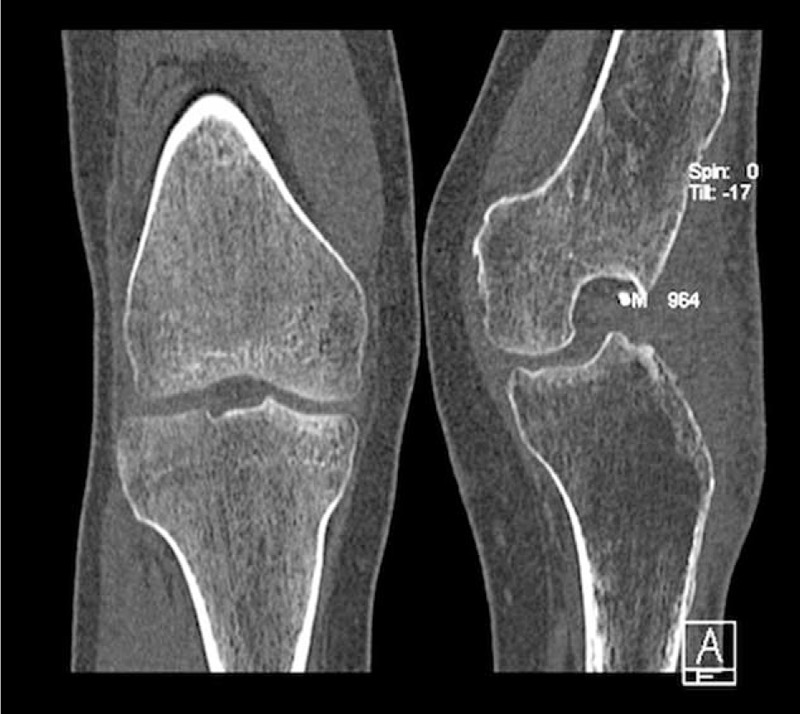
The computed tomography AP scan of both knees before arthrodesis.
